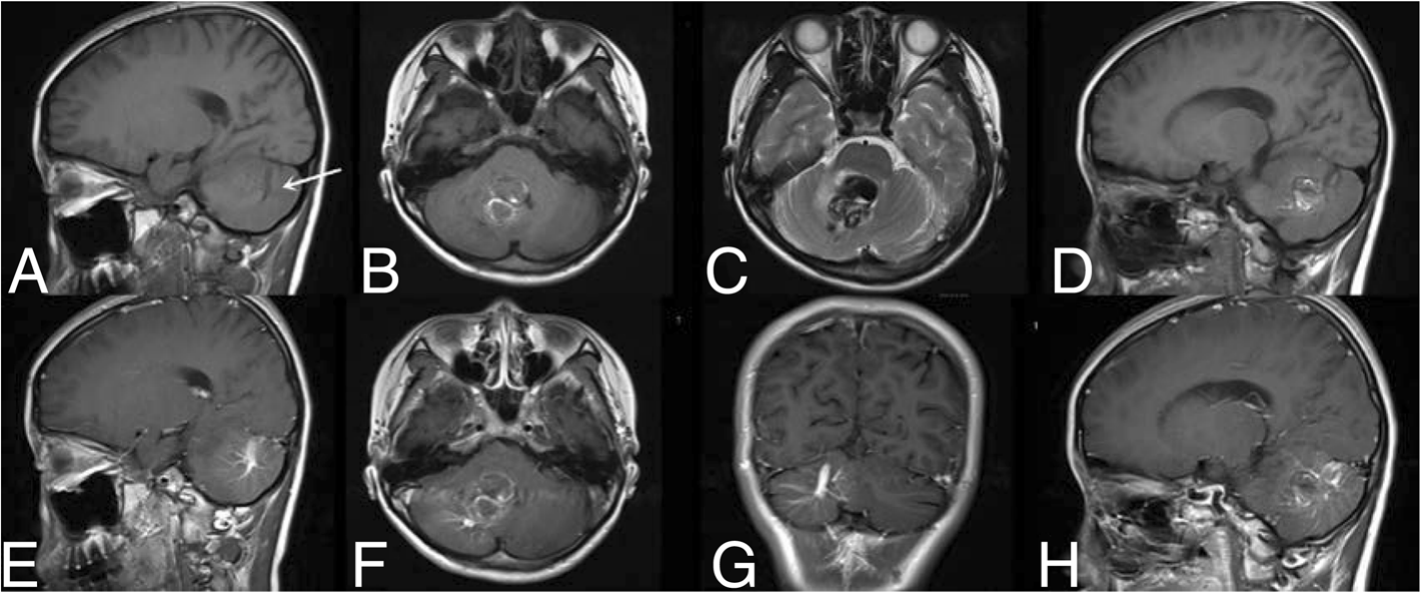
This is a 18-year-old female. It’s the typical MRI images for DVA coexist with CM. a b c d is the T1 weighted image, and e f g h is the enhanced image. The arrow refers to DVA
